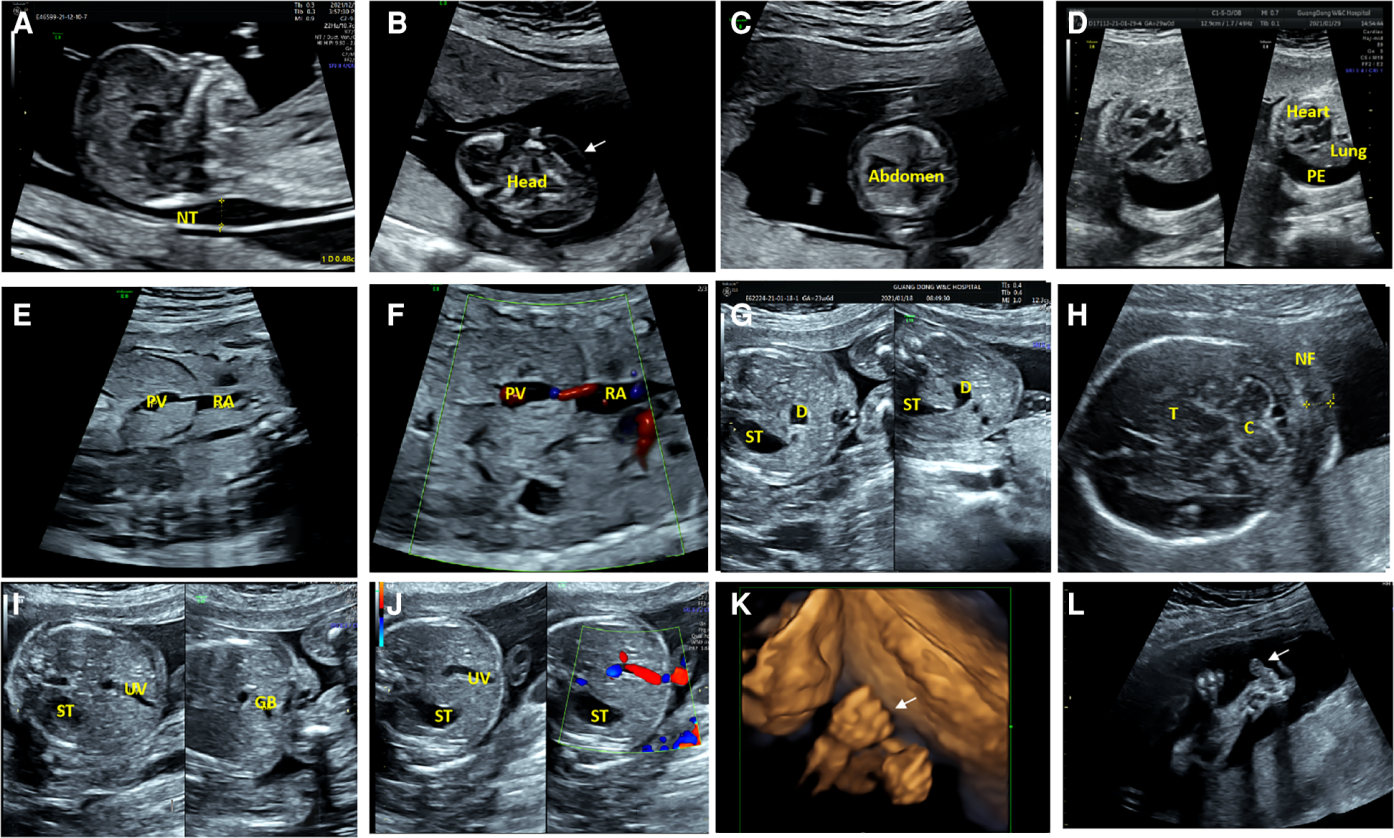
Less common ultrasound features in our study. (A) The ultrasonic examination of case 8 showed a thickened nuchal translucency (NT) in the midsagittal view. (B,C) The transverse view of the fetal brain and abdomen of case 8 showed hydroderma (the white arrow). (D) The ultrasound examination of case 10 showed a left pleural effusion (PE), and dextroposition of heart in the four-chamber view. (E,F) Two-dimensional and color Doppler ultrasonic images of case 9 showed the absence of venous duct and abnormal connection between the portal vein (PV) sinus and the right atrium (RA) in the oblique coronal view. (G)The transverse view of the upper abdomen of the fetus 11 presented a typical double bubble sign. The anechoic region on the left side of the abdomen is the stomach (ST) and the anechoic region on the right is the expanded duodenum (D). (H) The ultrasonic examination of the case 12 showed a thickened nuchal fold (NF) in the transverse view of cerebellar. C, Cerebellum; T, Thalamus. (I,J) Two-dimensional and color Doppler ultrasonic images of the fetus 11 showed that the umbilical vein(UV) runs towards to the stomach(ST), and the gallbladder(GB) is located to the left of the umbilical vein in the transverse view of the abdomen. (K) Three-dimensional ultrasonic images of the fingers showed the hands of case 12 presented in a continuous “Thumb-in-Fist Posture”. (L) The ultrasonic examination of case 12 showed that thumbs were continuously adducted, and hypoechoic mass presented in the left radial volar.
